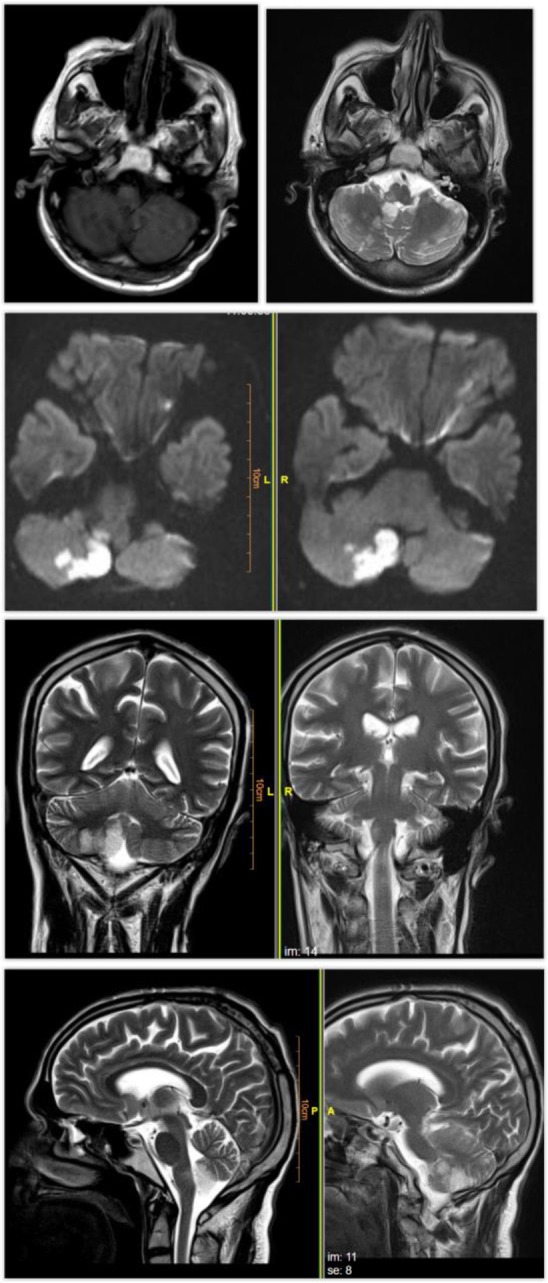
Brain MRI of the patient.
